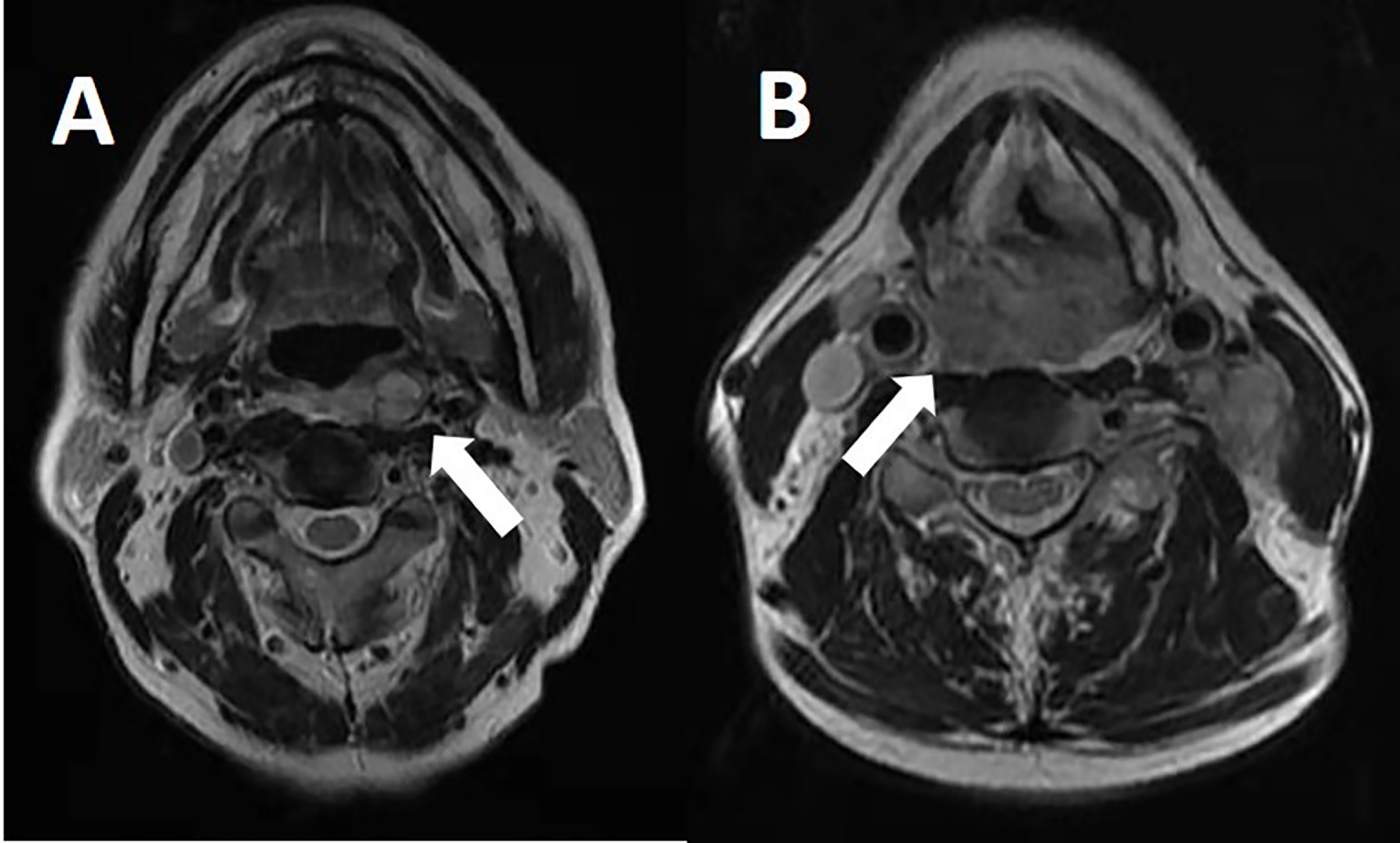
RPLN in MRI images. (A) Left RPLN metastasis in MRI. (B) Right RPLN metastasis in MRI.
